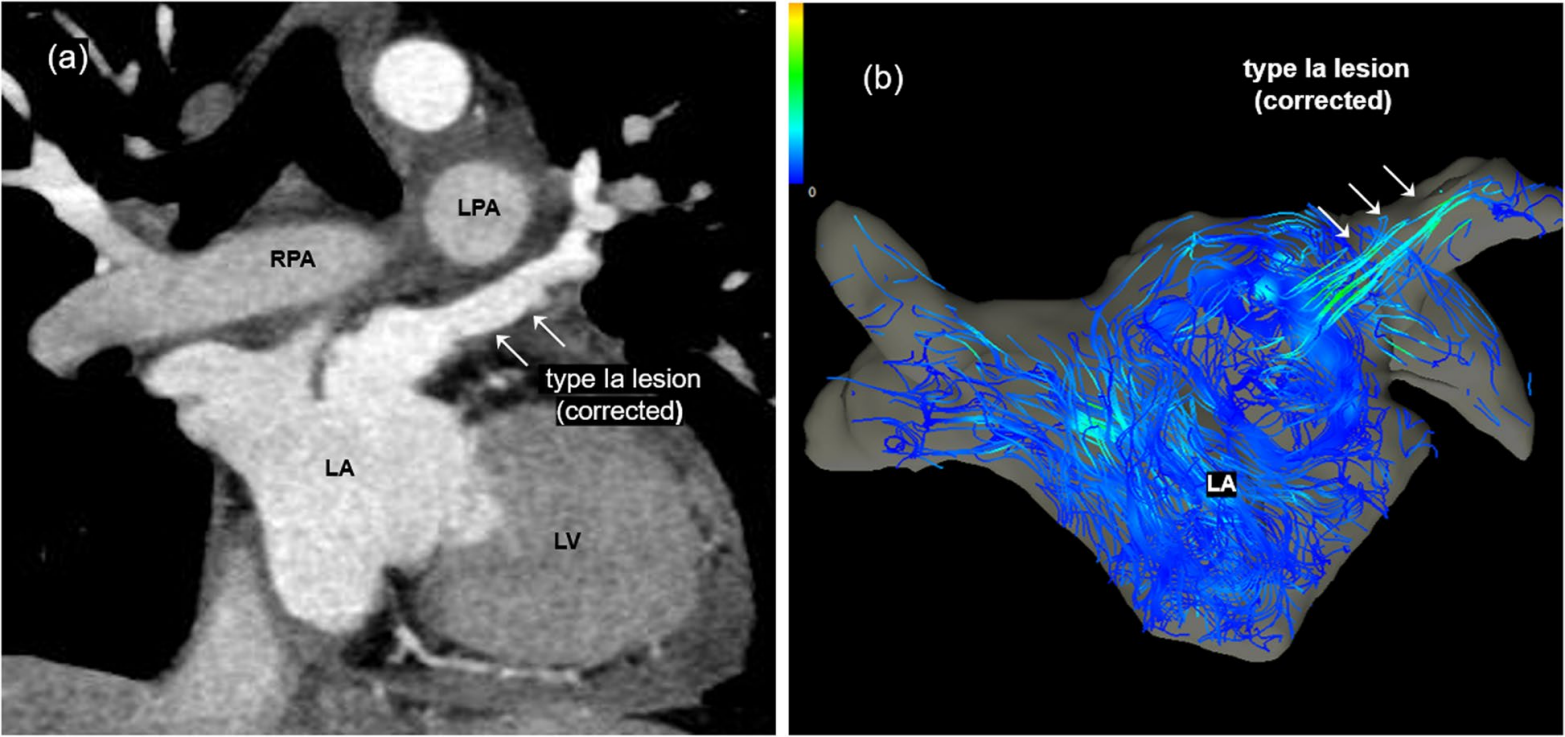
Postoperative computed tomography and 4D flow magnetic resonance imaging. a Coronal section of the postoperative computed tomography image demonstrates the repaired type Ia lesion without narrowing and kinking. b Postoperative 4D flow magnetic resonance imaging reveals a laminar flow through the corrected type Ia lesion. LA, left atrium; LPA, left pulmonary artery; LV, left ventricle
